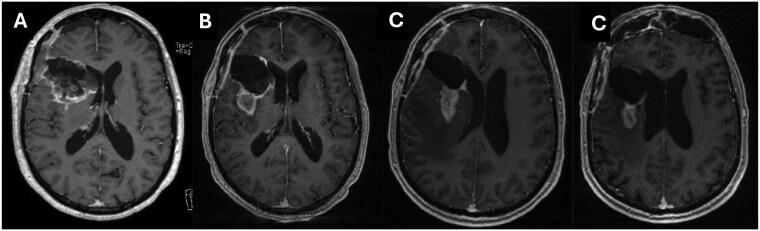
Brain MRI findings before and after receiving immune checkpoint inhibitor therapy. (A). Axial T1-post contrast MRI from a patient with newly diagnosed glioblastoma post-surgery and prior to initiation of 6-weeks of radiation therapy combined with durvalumab. (B). Axial T1-post contrast MRI 2 months after completion of combined radiation and ICI therapy, showing increase in nodular contrast enhancement. (C). Further increase in nodular enhancement at 2.5 months post radiation. (D). Decrease in nodular enhancement at 4 months post radiation, consistent with pseudoprogression.
